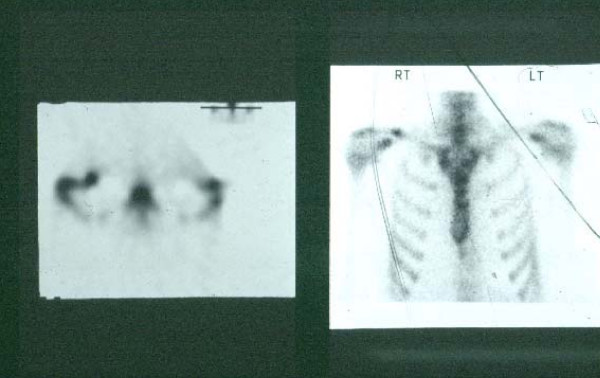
Single photon emission computed tomography scan of shoulders. A single photon emission computed tomography scan showing increased uptake in the lateral clavicle consistent with the site of pain.
